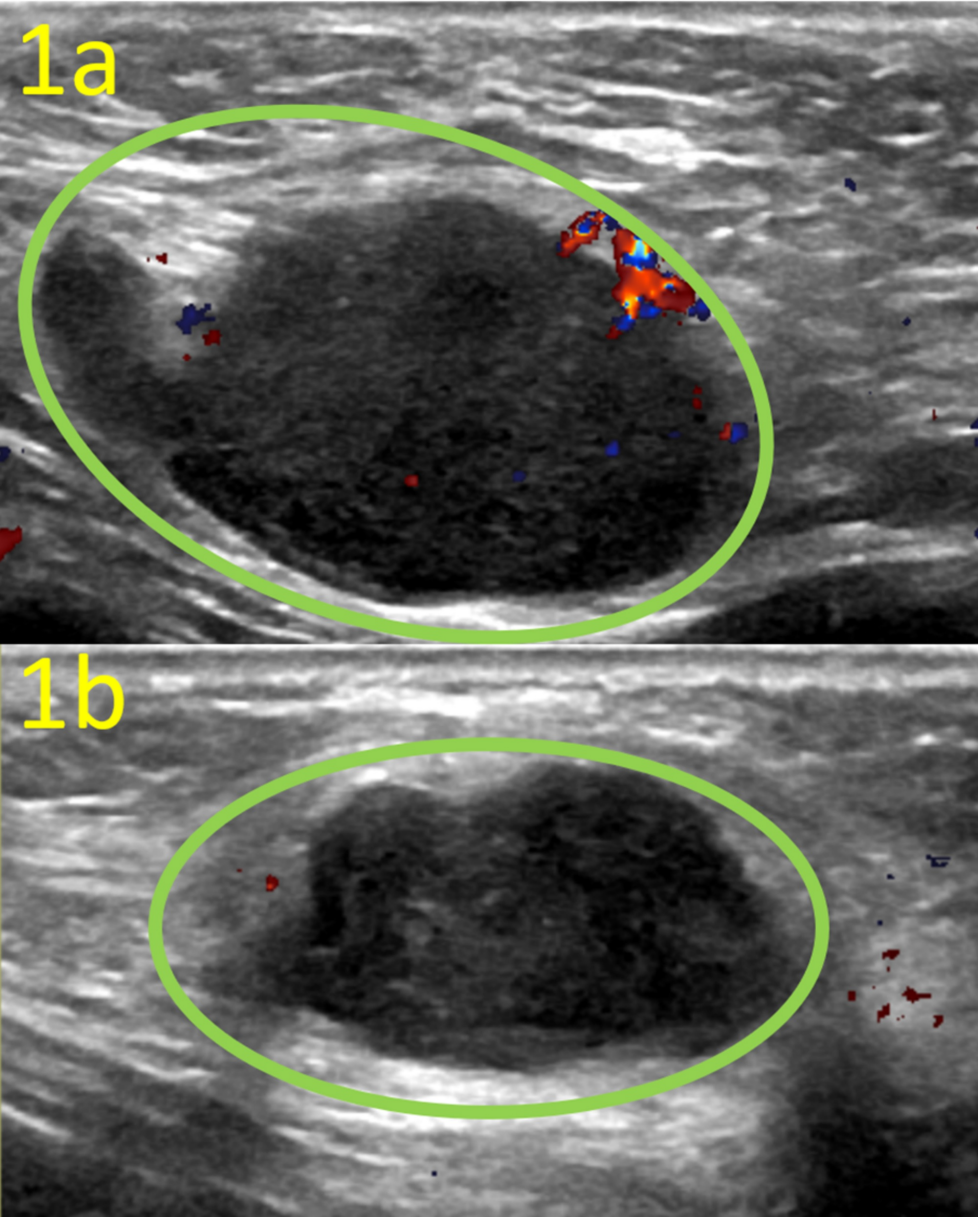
Targeted Ultrasound of the Bilateral Low Axilla These ultrasound images of the bilateral low axilla demonstrate morphologically abnormal lymph nodes (green circles) with loss of fatty hilum and cortical thickening. Left axillary lymph node (a) measures 35 x 19 x 31 mm; right axillary lymph node (b) measures 27 x 13 x 20 mm.
